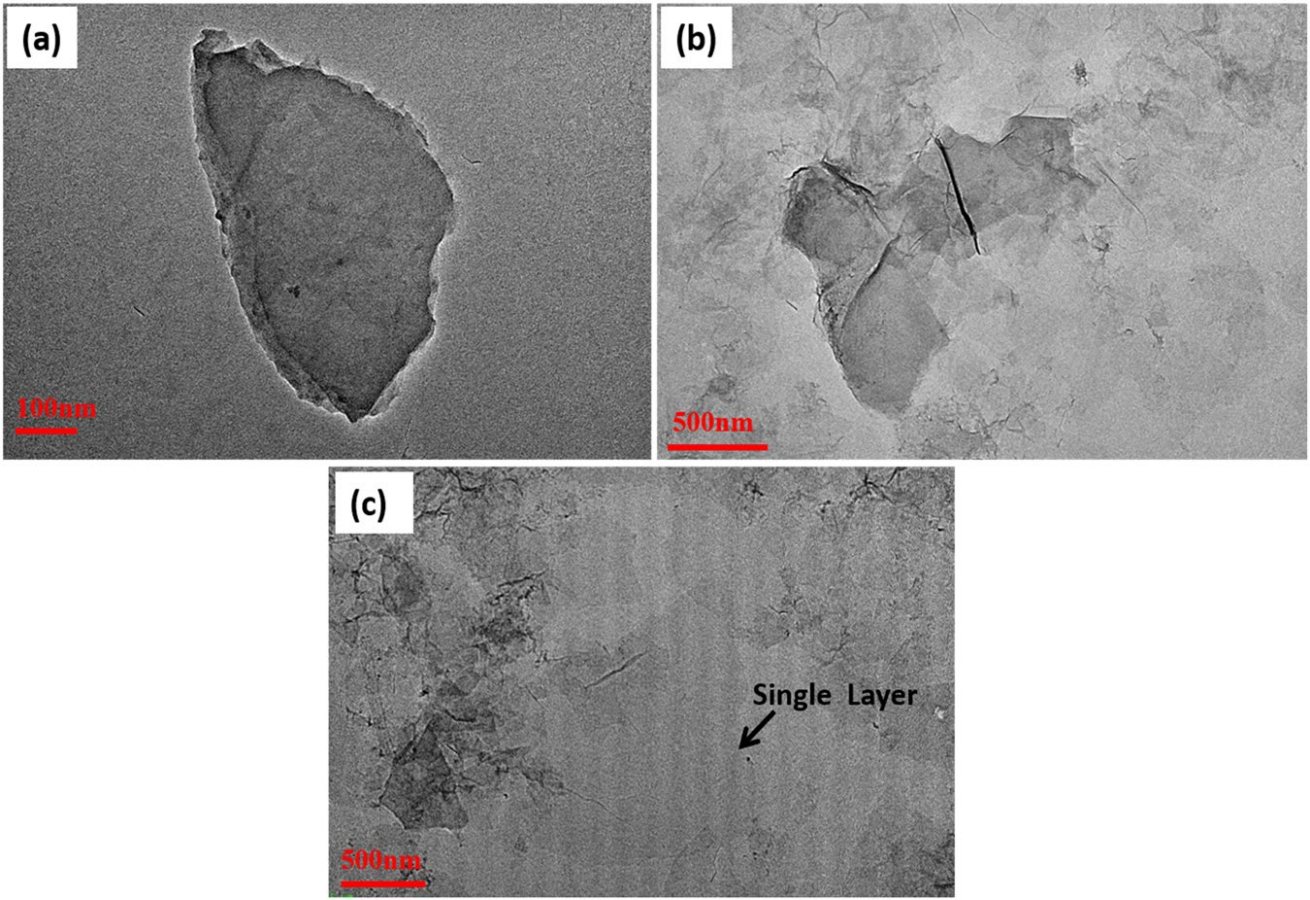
HRTEM images of GO as a function of ultrasonication time (a) 1 h, (b) 3 h, and (c) 5 h.
